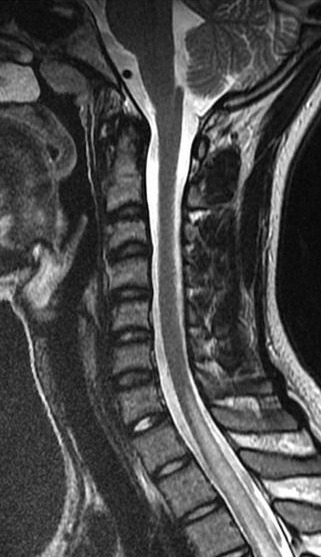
MRI spine showing hyperintensity in the thoracic cord till T12 level (2)
